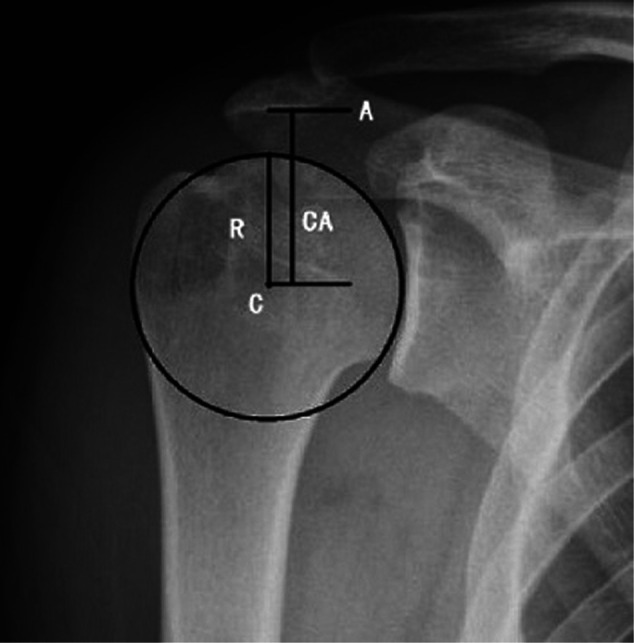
Radius of the humeral head (*R*) and CA distance were measured on an X-ray. UMI = CA/*R*.
